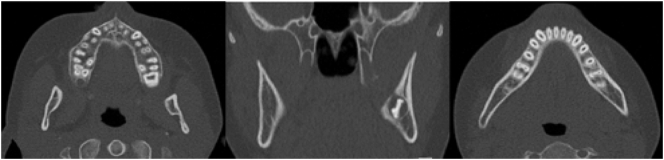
CT scan six months after the last injection of triptorelin showed completely restored anatomy and a total regress of the osteomyelitis in the right mandible.
